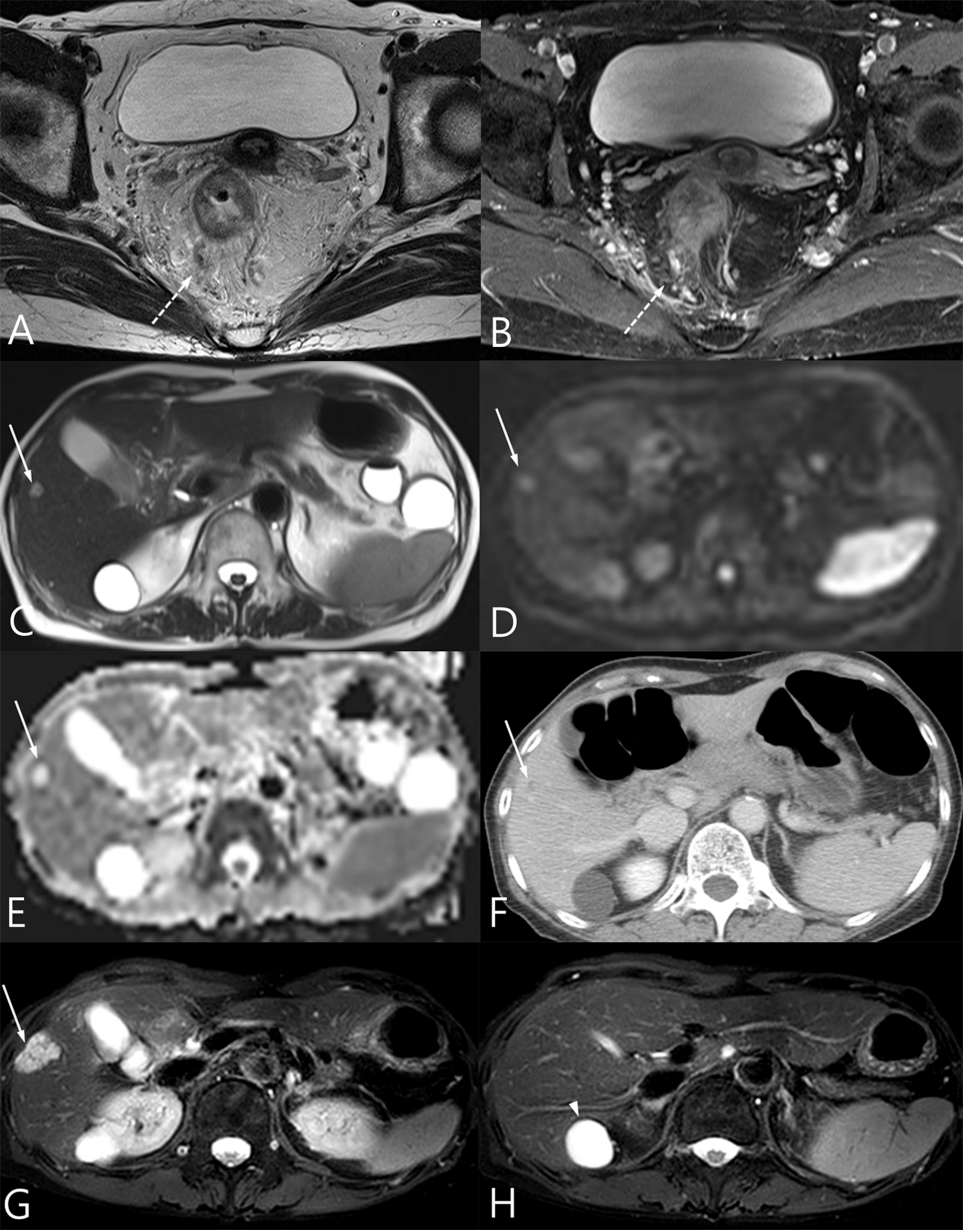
A 65-year-old female diagnosed with distal rectal cancer. (A) Perivascular stranding around the small vessels with a signal void on T2WI was noted. (B) Vessels around the rectum show contrast filling after contrast injection. (C) A small nodule with high signal intensity on T2WI is noted in segment five. (D-E) The lesion shows high signal intensity on DWI (b = 900 s/mm^2^) (D) without diffusion restriction on the ADC map (E). (F) The nodule shows focal peripheral enhancement in segment five on CT. The lesion was considered to be a hemangioma. (G) At the 2-year follow up, the lesion appeared to be enlarged on T2WI liver MRI after the patient underwent a laparoscopic abdominal transanal proctosigmoidectomy with coloanal anastomosis. The lesion was confirmed as liver metastasis of mucinous adenocarcinoma after liver segmentectomy. (H) Another lesion with high signal intensity on segment one on T2WI was also resected and diagnosed as a biliary cyst.
